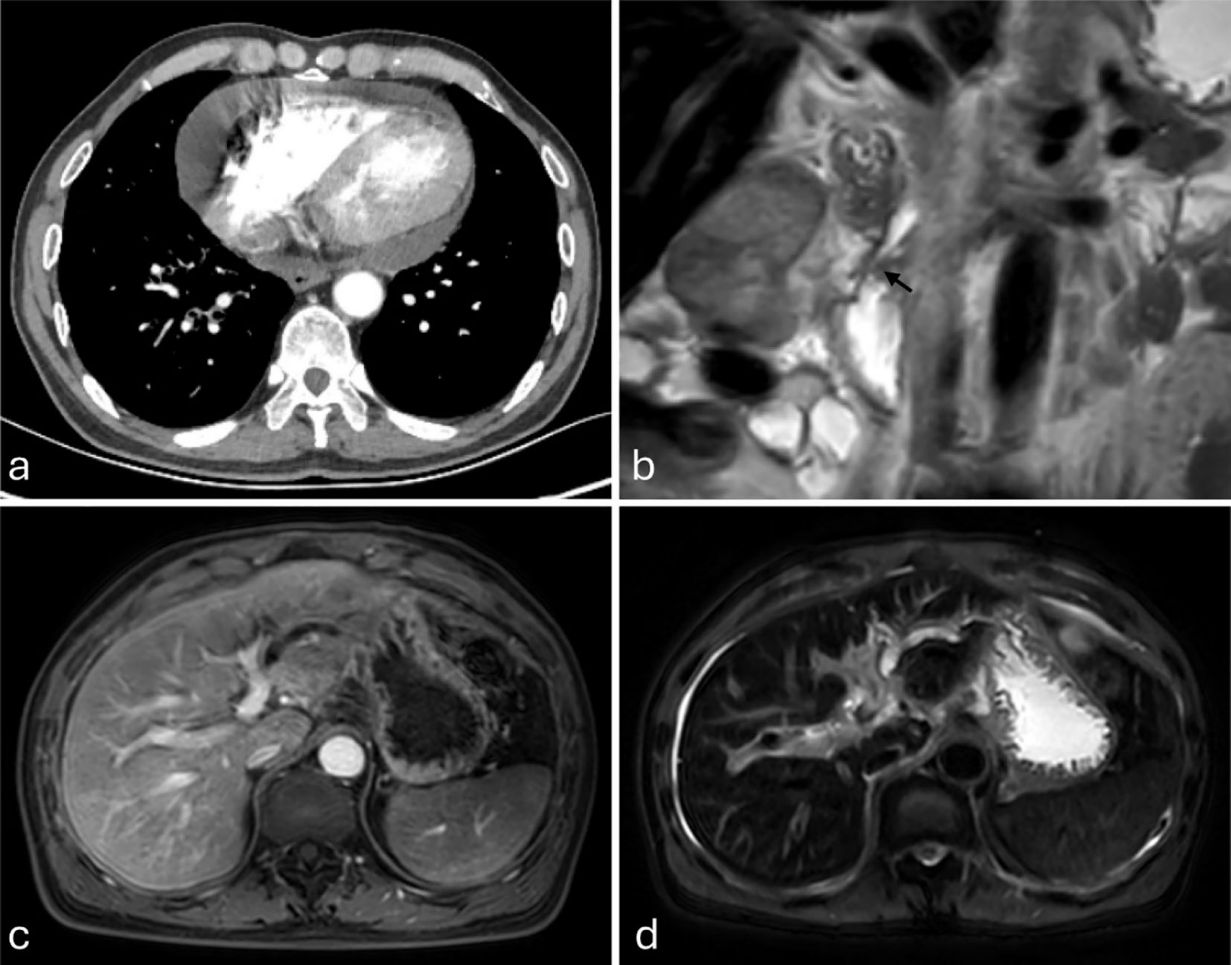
(a–d) Imaging studies from an external institution (December 5, 2024). (a) Contrast-enhanced chest CT demonstrates pericardial effusion. (b–d) Magnetic resonance imaging (MRI), (b) Coronal T2-weighted imaging (T2WI) clearly demonstrates tapered stenosis at the distal common bile duct and pancreatic duct orifice (marked by black arrows). (c) Axial fat-suppressed contrast-enhanced T1-weighted imaging (T1WI) shows intrahepatic biliary dilatation in the left hepatic lobe. (d) Axial fat-suppressed T2WI demonstrates left hepatic lobe intrahepatic biliary dilatation and ascites.
